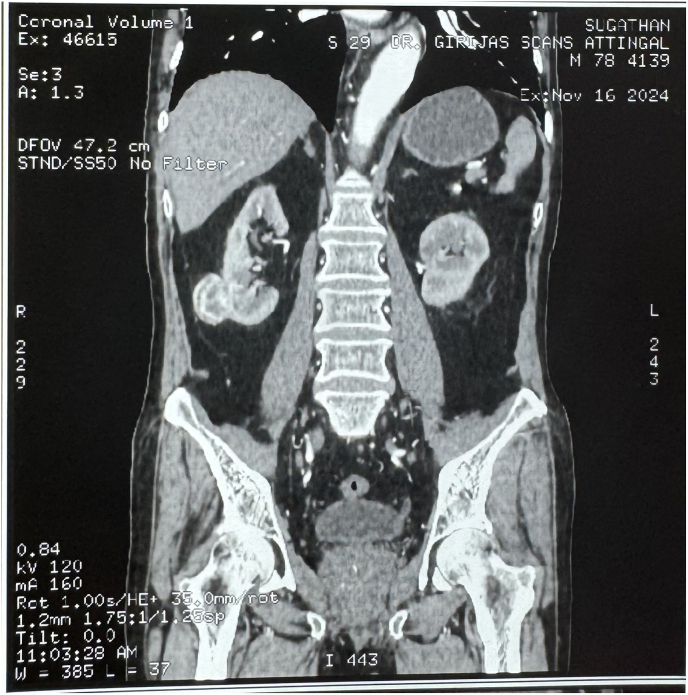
Computed tomography showing a well-defined hypo-to iso-dense exophytic lesion measuring 29 × 30 × 35 mm arising from the posterolateral aspect of the lower pole of the right kidney.
